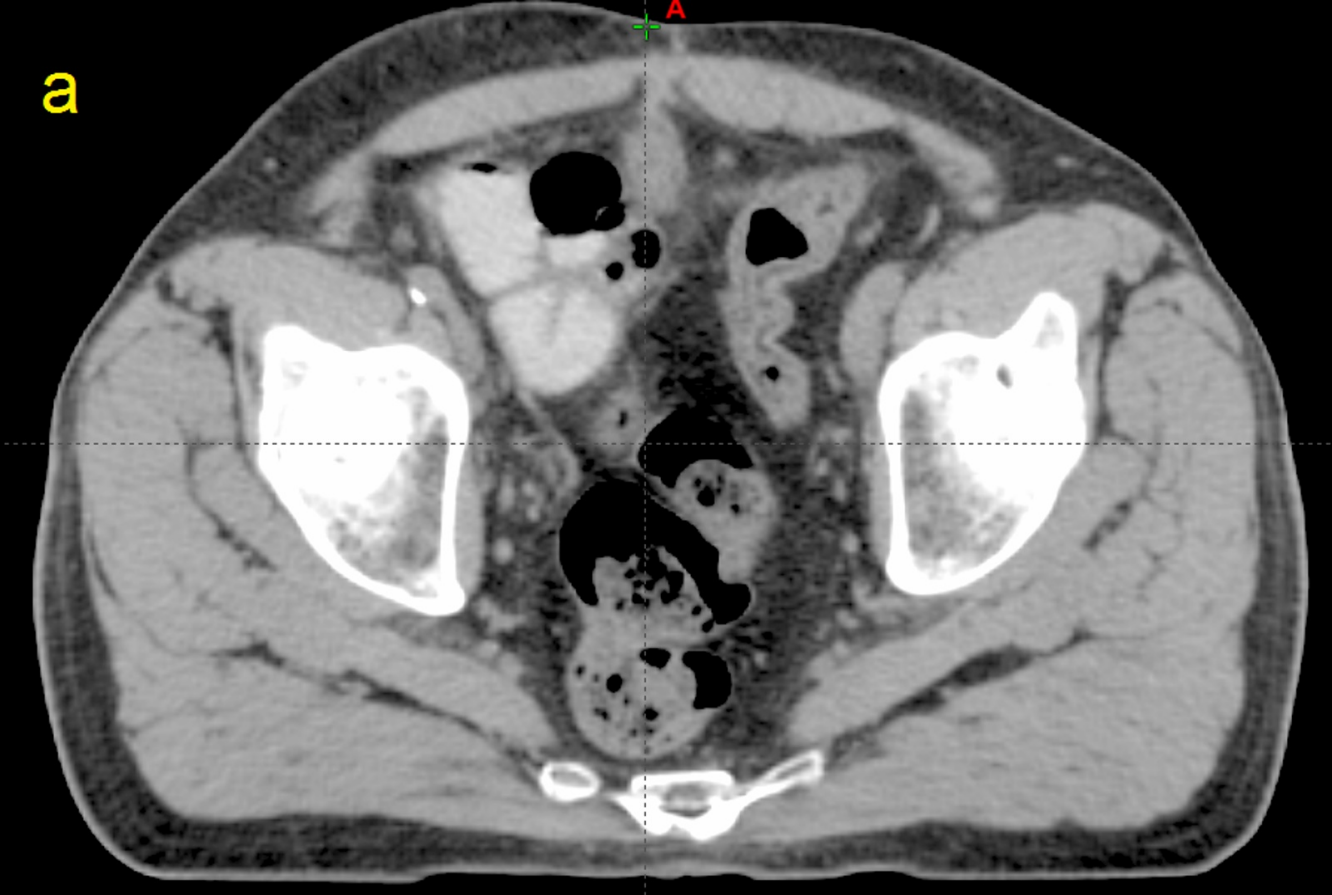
Computed tomographic (CT) image taken from patient P12 a) A slice from the planning CT scan.
